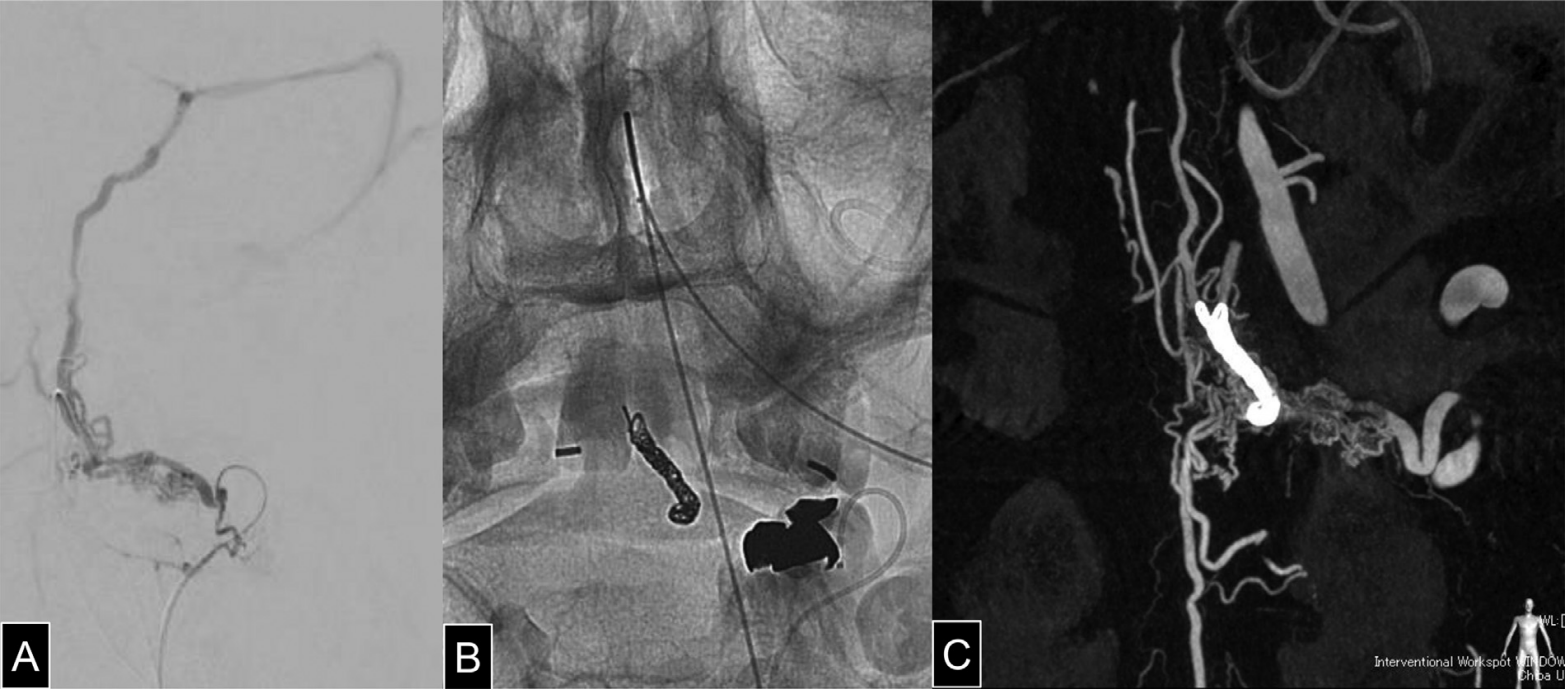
(A) Selective angiography of the radiculomeningeal artery arising from the left vertebral artery demonstrates venous drainage reaching the anterior lateral spinal vein via the internal jugular vein and superior petrosal sinus. (B) The left anterior lateral spinal vein was embolized with 7 coils via a transvenous approach. (C) Cone-beam CT performed via the left vertebral artery after coil embolization reveals persistent perimedullary venous enhancement in the arterial phase, indicating incomplete occlusion of the shunt.
